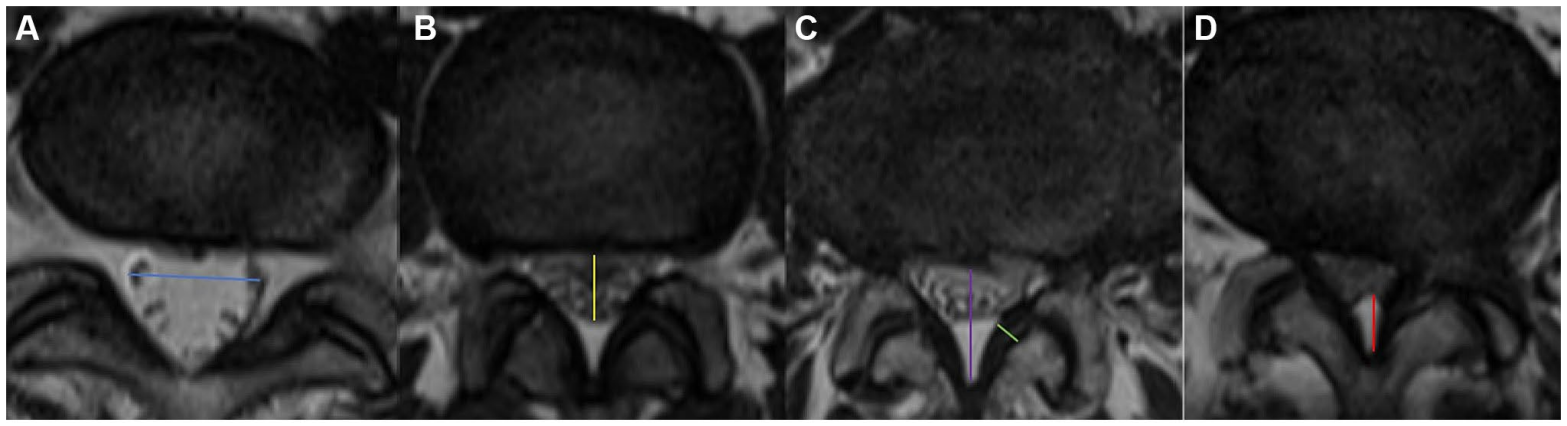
Visualization of the measurement of blue arrow the TDD (a), yellow arrow the APDD (b), purple arrow the APDS (c), green arrow the LF thickness, and red arrow EF. Axial appearance of EF on T2-weighted MR images. The degree of fat accumulation at each intervertebral disc level was classified as follows: absent (a), concave (b), flat (c), or convex (d).
